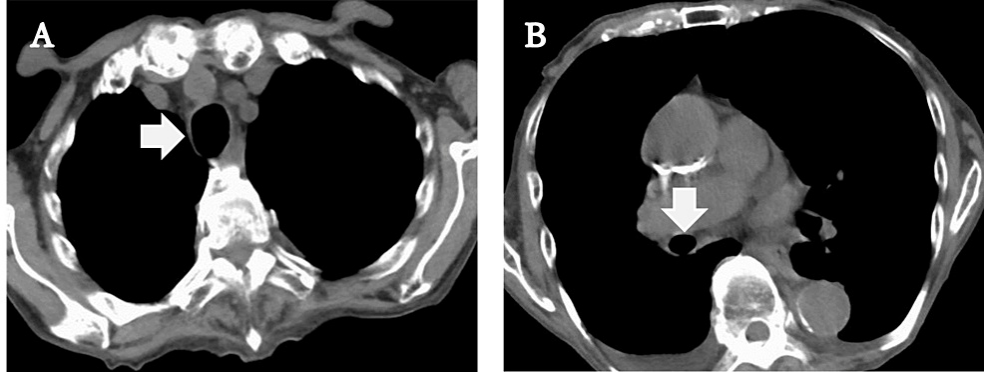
Post-treatment plain CT. A is the central side, and B is the peripheral side from the bifurcation. Bronchial wall thickening had disappeared. The central bronchus, which had been lengthened in the anterior-posterior direction, was also improved and became a round form.
